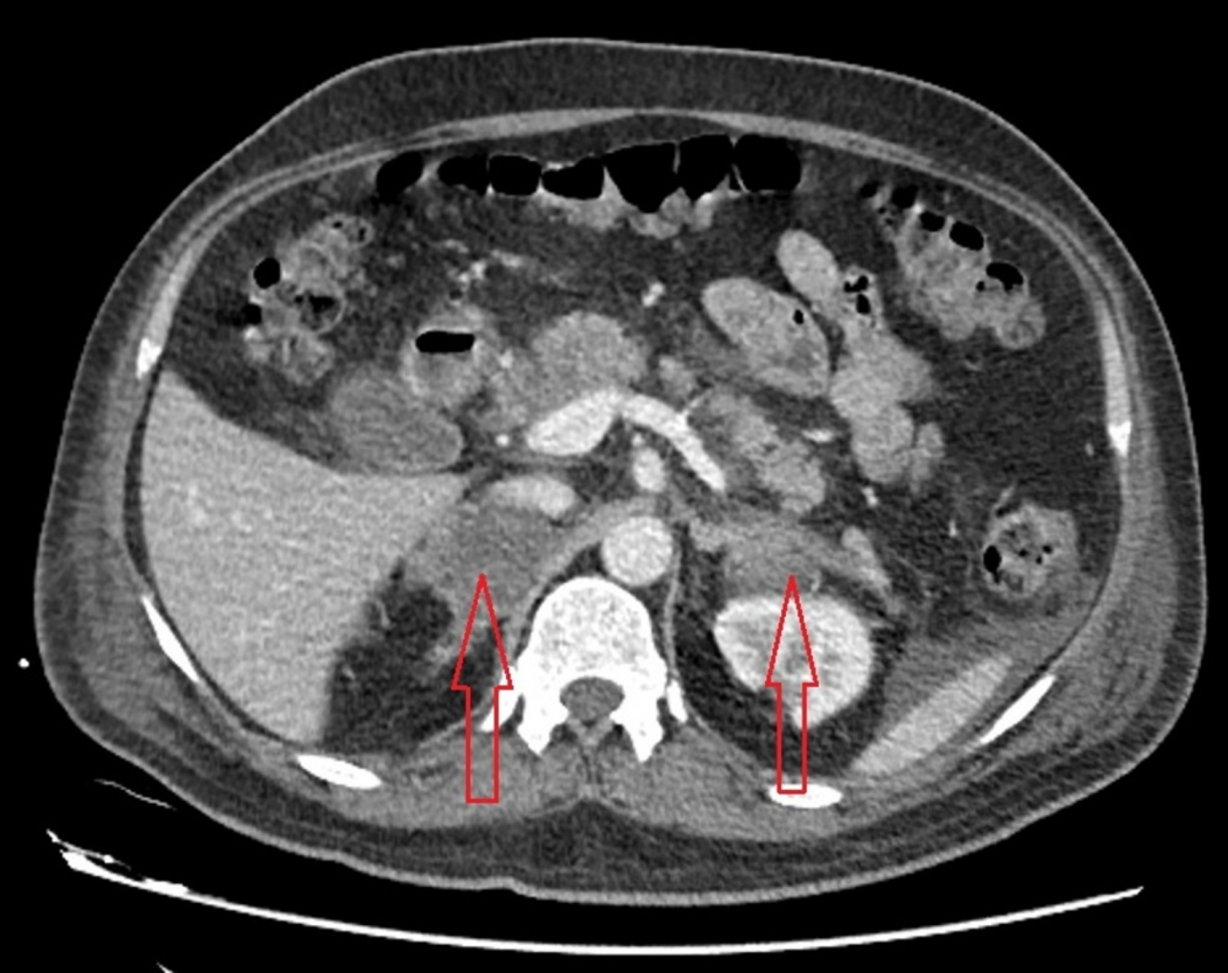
Contrast-enhanced computed tomography (CT) scan of the abdomen Red arrows: Enlarged right adrenal gland with a hetrogenous hyperdense area. A similar hetrogenous lesion is present in lateral limb of left adrenal gland.
